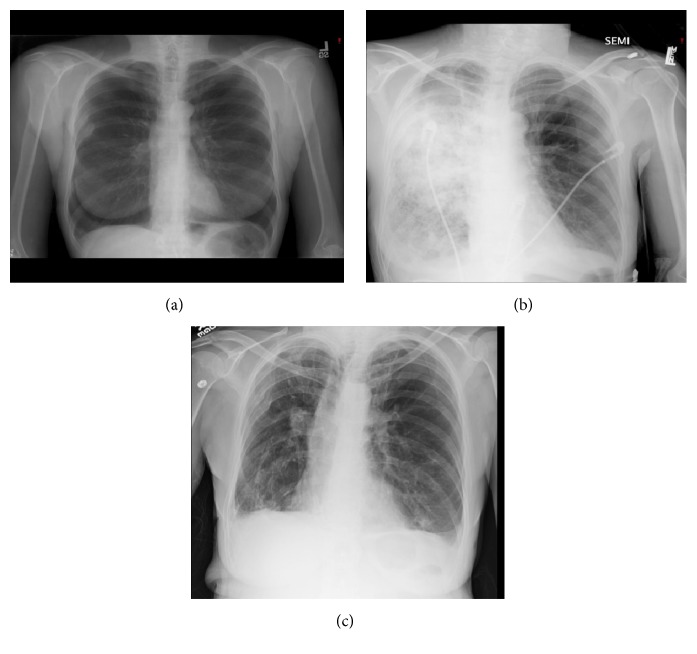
Baseline (a), during pseudomonas pneumonia (b), and follow-up (c) chest x-rays.
